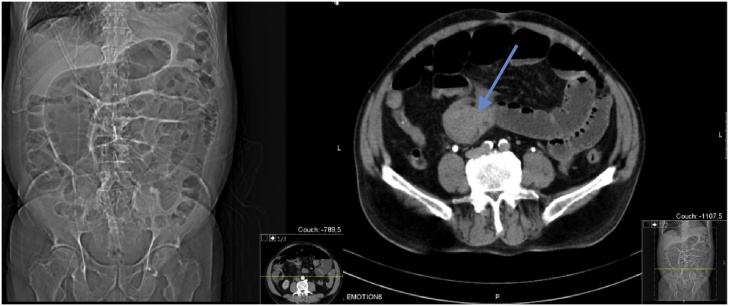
Preoperative computed tomography scan showing small bowel mass (arrow) causing intestinal dilatation and obstruction.
